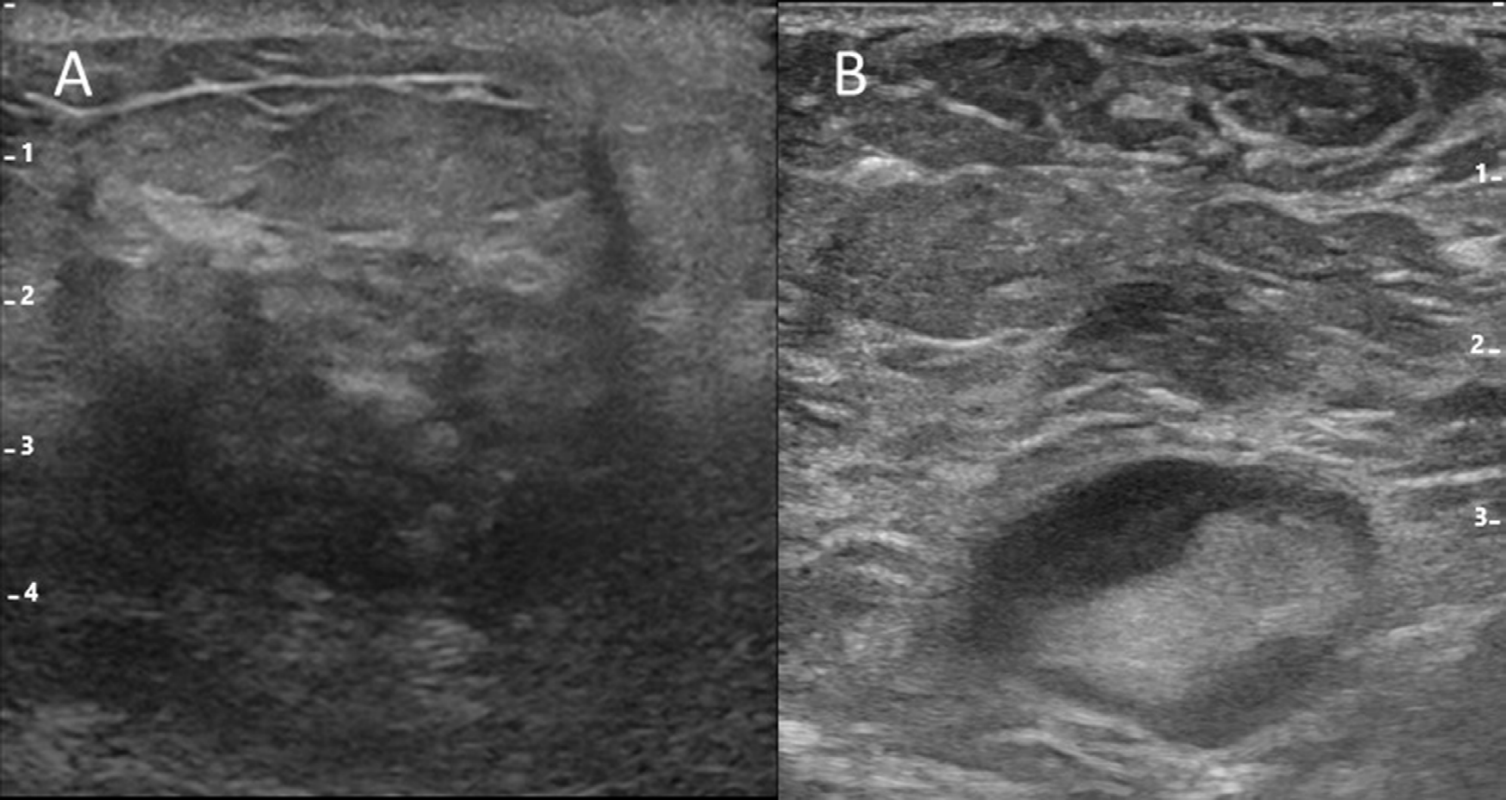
B-mode ultrasound. B-mode ultrasound shows an irregularly shaped mass of 50 mm in the right breast (A). In addition, there were multiple enlarged lymph nodes with irregularly thickened cortices in the right axilla (B).
